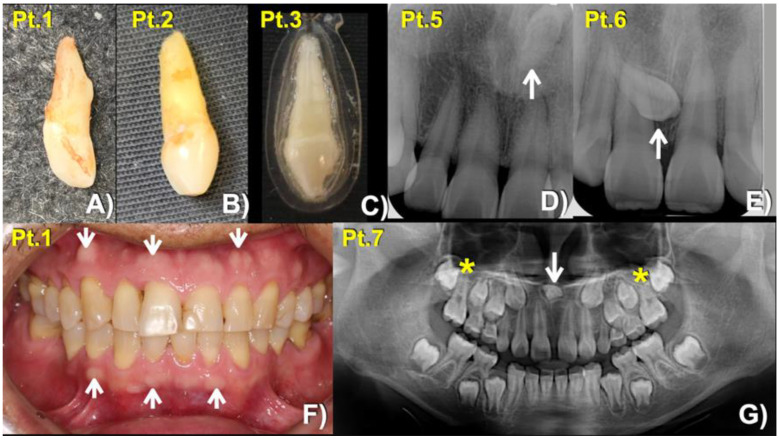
Mesiodens phenotypes. Extracted mesiodens of (A) patient 1, (B) patient 2. (C) patient 3. (D,E) Periapical radiographs show (D) Patient 5—Inverted mesiodens (arrow). (E) Patient 6—Unerupted mesiodens (arrow). (F) Patient 1—Buccal exostoses (arrows). (G) Panoramic radiograph showing inverted mesiodens (arrow) and unseparated roots of the maxillary first permanent molars (asterisks).
